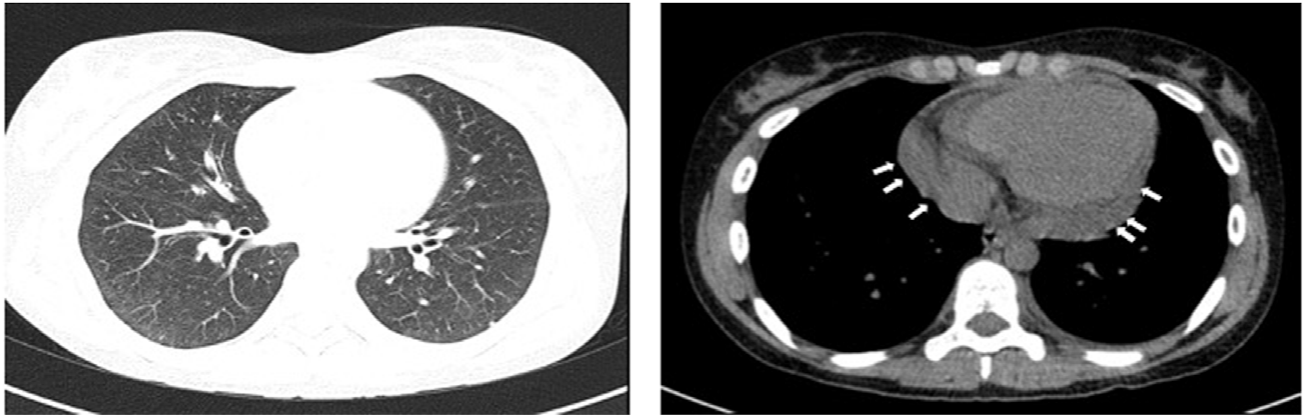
Thorax CT scan shows interstitial lung thickening with peripheral opacities. Thorax CT scan shows small pericardial effusion, as indicated by white arrows.
